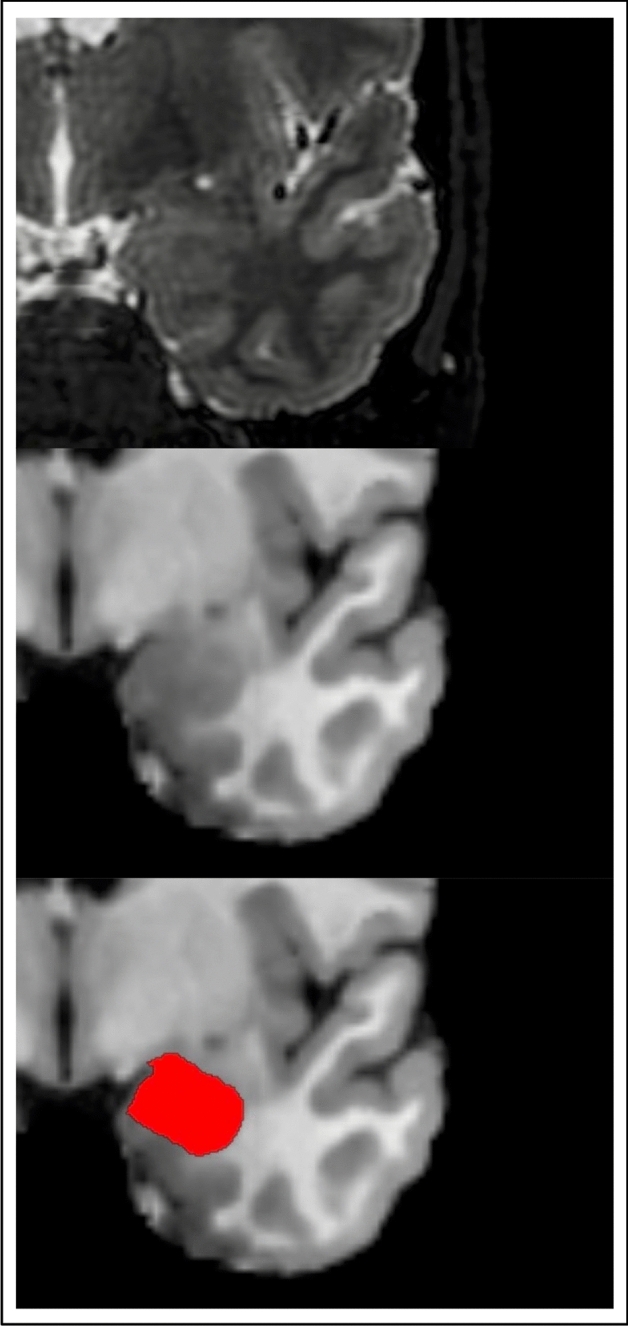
Segmentation of the amygdala. T2-weighted and T1-weighted images of the amygdala as segmented by FreeSurfer.
